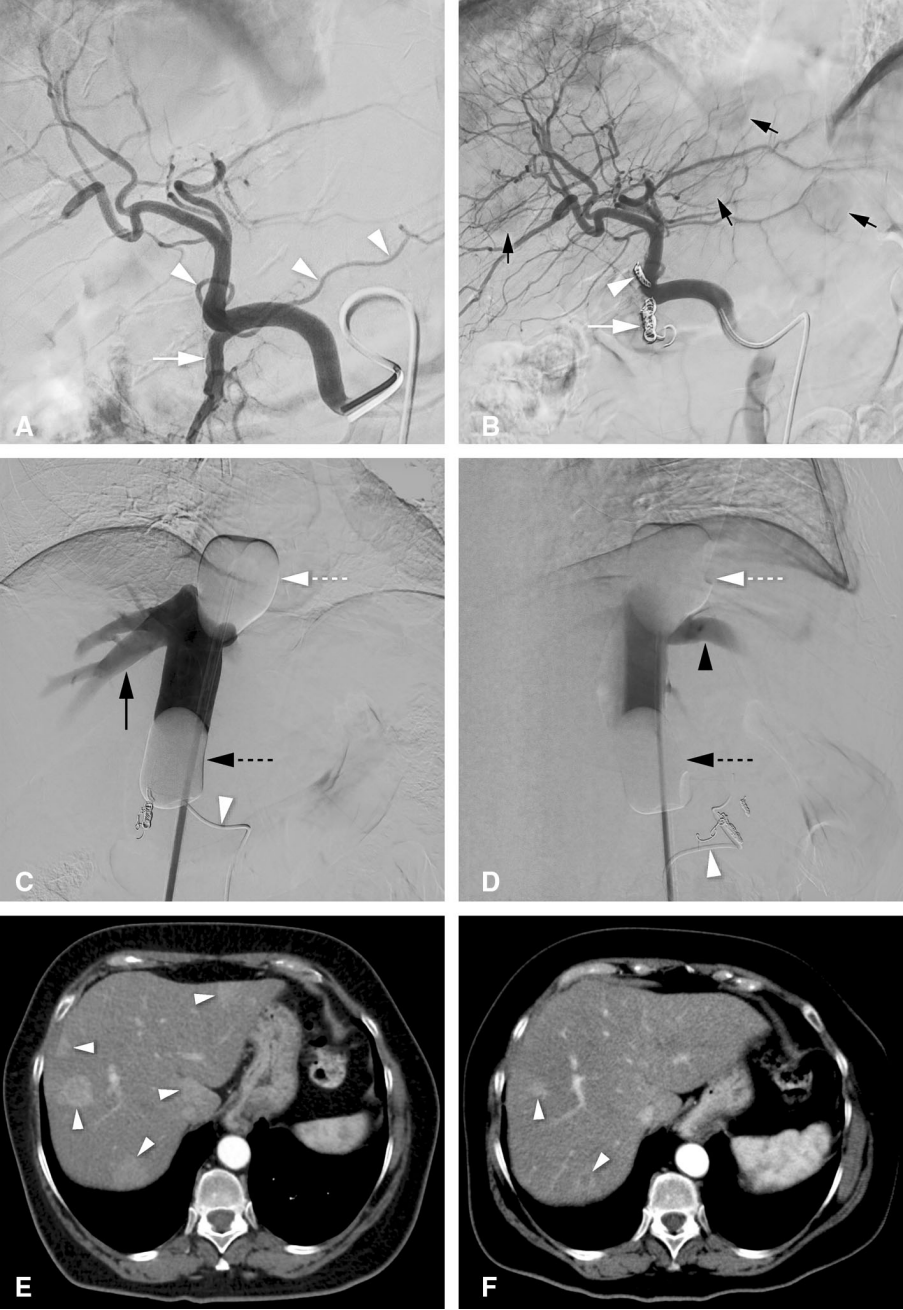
Hepatic vascular mapping and M-PHP in a 59-year-old female with bilobar hepatic metastases from uveal melanoma. A Angiographic image from the celiac trunk, showing a right gastric artery (white arrowheads) and gastroduodenal artery (white arrow) from the common hepatic artery. B Successful coiling of the right gastric artery (white arrowhead) and gastroduodenal artery (white arrow). Multiple hypervascular metastases are seen in both liver lobes (black arrows). C, D Posteroanterior and lateral images during venography, performed by manual injection of non-diluted contrast medium through side holes of the double-balloon catheter. The cranial balloon (dotted white arrow) was inflated at the atriocaval junction and the caudal balloon (dotted black arrow) in the infrahepatic portion of the inferior vena cava. Note the opacification of the right hepatic vein (black arrow) and middle hepatic vein (black arrowhead), while there was no leakage alongside the balloons. A microcatheter (white arrowhead) was placed into the hepatic artery proper for the infusion of melphalan. E Axial CT image in arterial phase before treatment showing five hepatic metastases (white arrowheads). F Axial CT image in arterial phase after two cycles of M-PHP showing reduction in size of two metastases in the right lobe. The other three metastases showed a complete radiological response
